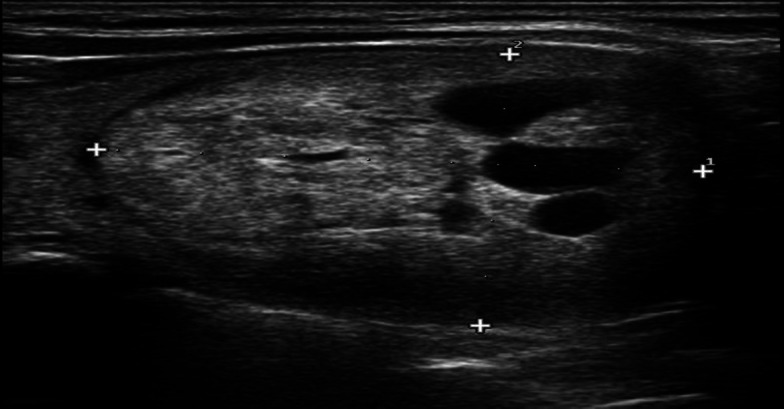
Ultrasonographic appearance of TI-RADS III nodule; they are solid or almost solid, iso-, or hyperechoic, well-defined oval shape nodule without echogenic foci
